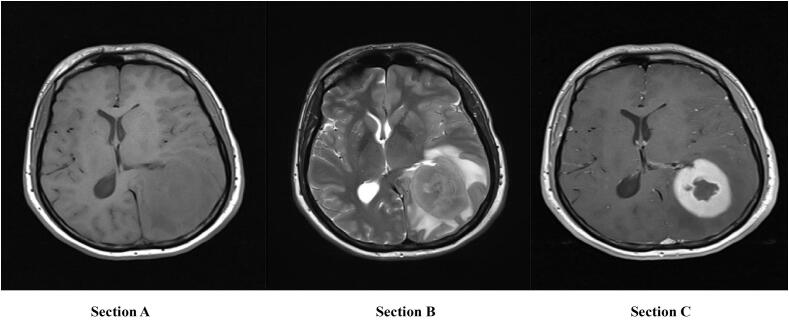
Magnetic resonance imaging (MRI) axial sections with no contrast in T1-weighted imaging (WI) (A); T2-weighted imaging (B) and with contrast in T1 WI (C) showing a left intraaxial parieto-occipital lesion.
